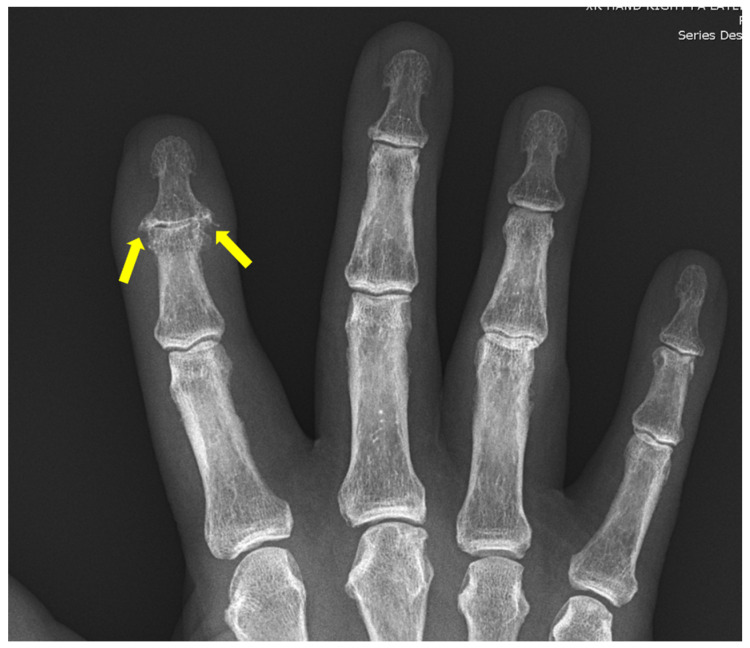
79-year-old male with psoriatic arthritis. Hand radiographs demonstrate diffuse soft-tissue swelling in the index finger (sausage finger). Small marginal erosions are present in the DIP joint along with mild fluffy periosteal reaction.
